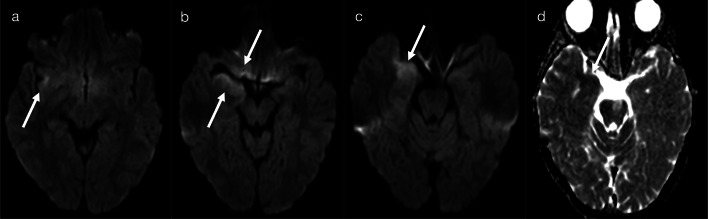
Restricted diffusion in supratentorial grey matter—mesial temporal lobe (Pattern 2B). 7-year-old child with headache, fluctuating consciousness and tonic–clonic seizures. CSF was positive for HSV-1. DWI (a–c) and ADC map (d) demonstrated asymmetric signal abnormalities predominantly involving the right mesial temporal lobe (amygdala and uncus), anterior perforated substance and insular cortex (arrows), as would be typical for HSV encephalitis in adults
